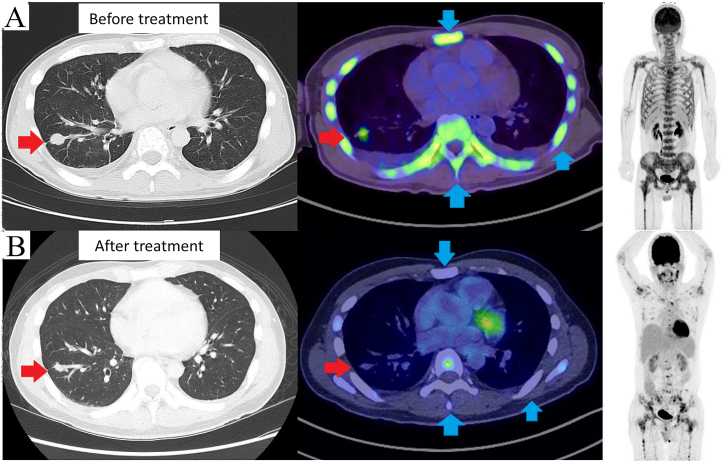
CT and PET/CT scans before treatment (A) show diffusely high FDG uptake, including the mass in the right lower lobe (red arrow), vertebral body, sternum, and ribs (blue arrow). Six months after starting treatment with gefitinib (B), CT and PET/CT scans showed that the mass in the right lower lobe had shrunk, and the diffuse bone metastases had little or no FDG uptake.
